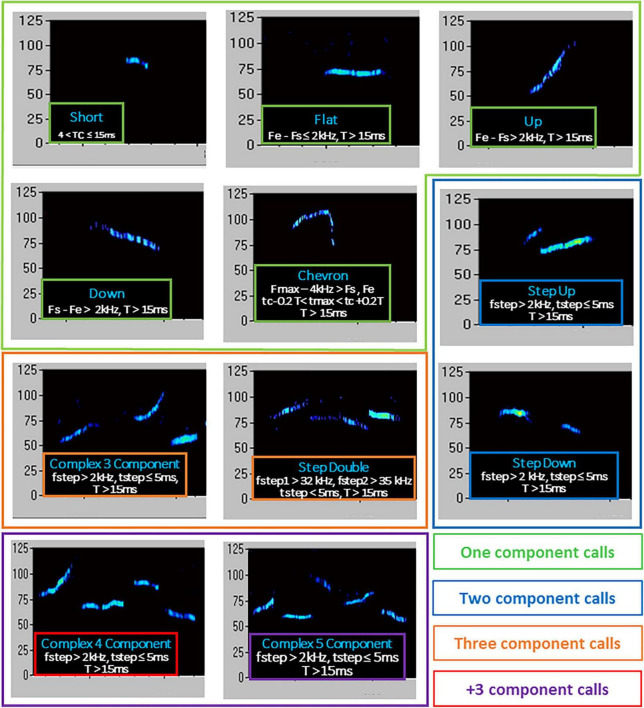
Type of ultrasonic calls evaluated in the study. T, duration of call; Fs, start frequency; Fe, end frequency; Fmax, highest frequency; Fmin, lowest frequency; fstep, frequency step; tmax, time of max frequency; tmin, time of min frequency; tc, time at center of call. Call types were automatically classified using the software Sonotrack, based on the parameters described here. Definitions of call types were mutually exclusive. Overlap of components was removed when more than 70% to prevent wrong call durations. Short gaps between components in both frequency (≤6 kHz) and time (≤5 ms) were interpolated (gaps can be caused by changes in microphone sensitivity or direction of vocalization). The calls classified as “complex 3 component” and “+3 component” were summed up into a “total complex” category.
